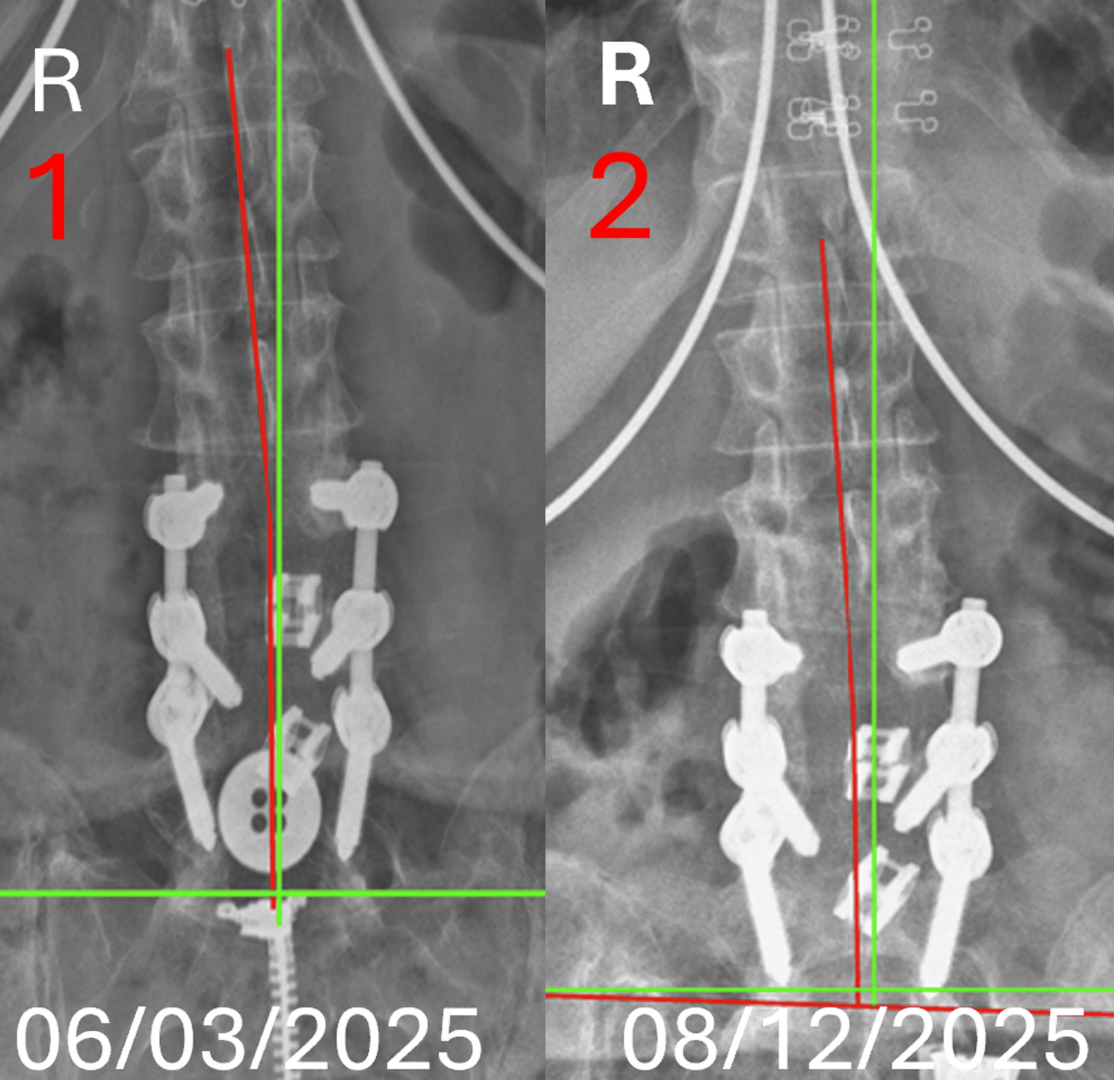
Anteroposterior (AP) lumbopelvic radiograph comparison The green line depicts the expected alignment of the posterior longitudinal ligament in a normal spine. The red line represents the subjects' alignment as defined by George’s line, reflecting deviation of the posterior longitudinal ligament. (1) X-ray image represents initial presentation to the office on 06/03/2025. Pre-treatment AP lumbar radiograph demonstrating translation of T12-S1 of -8.3 mm (normal is measured 0.0 mm). (2) X-ray image represents the day of discharge from the office on 08/12/2025. Post-treatment AP lumbar radiograph demonstrating translation of T12-S1 of -7.3 mm (normal is measured 0.0 mm).
